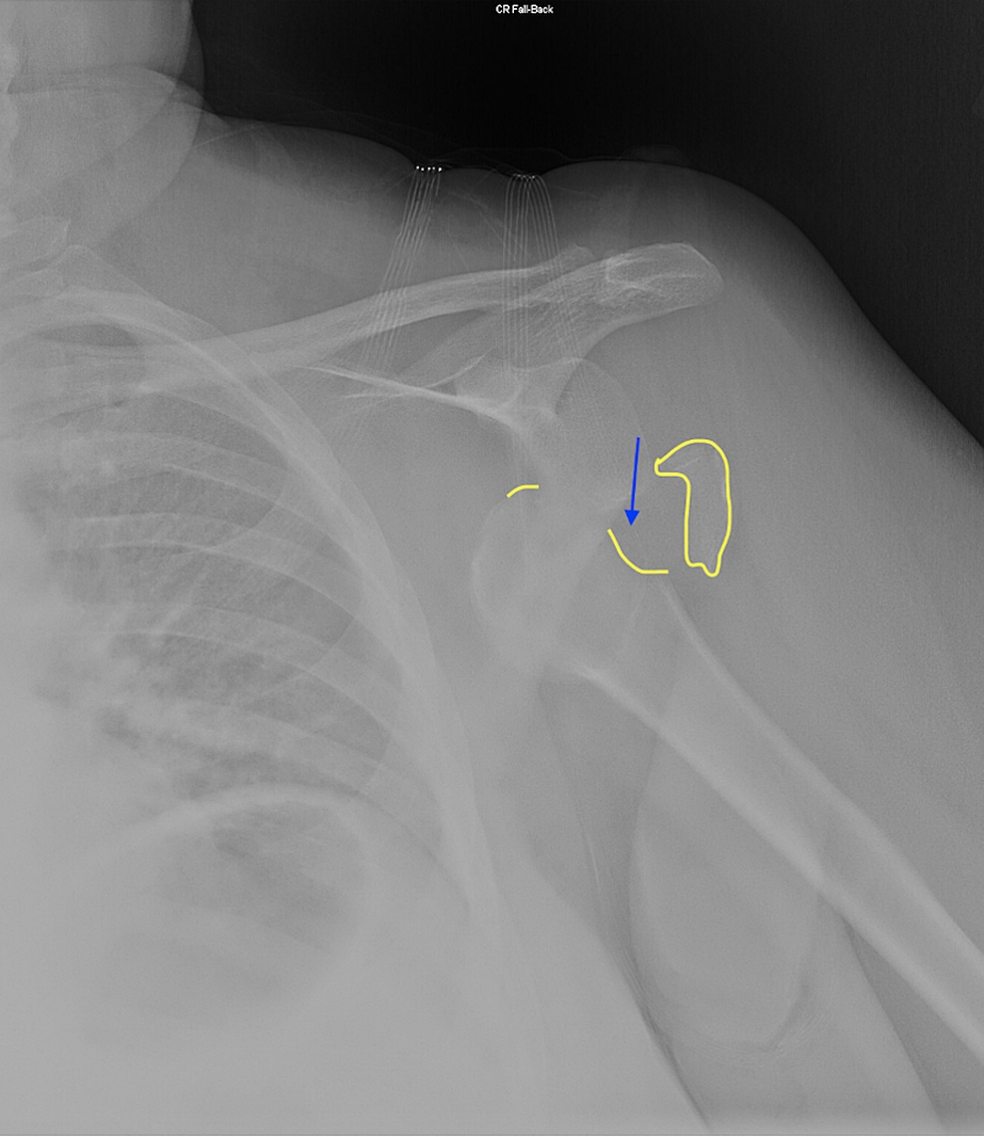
Patient’s shoulder radiograph pre-reduction with an outline of the Hill-Sachs fracture and an arrow pointing toward the Hill-Sachs lesion on the humeral head. Outlined in yellow is a loose fracture fragment.
